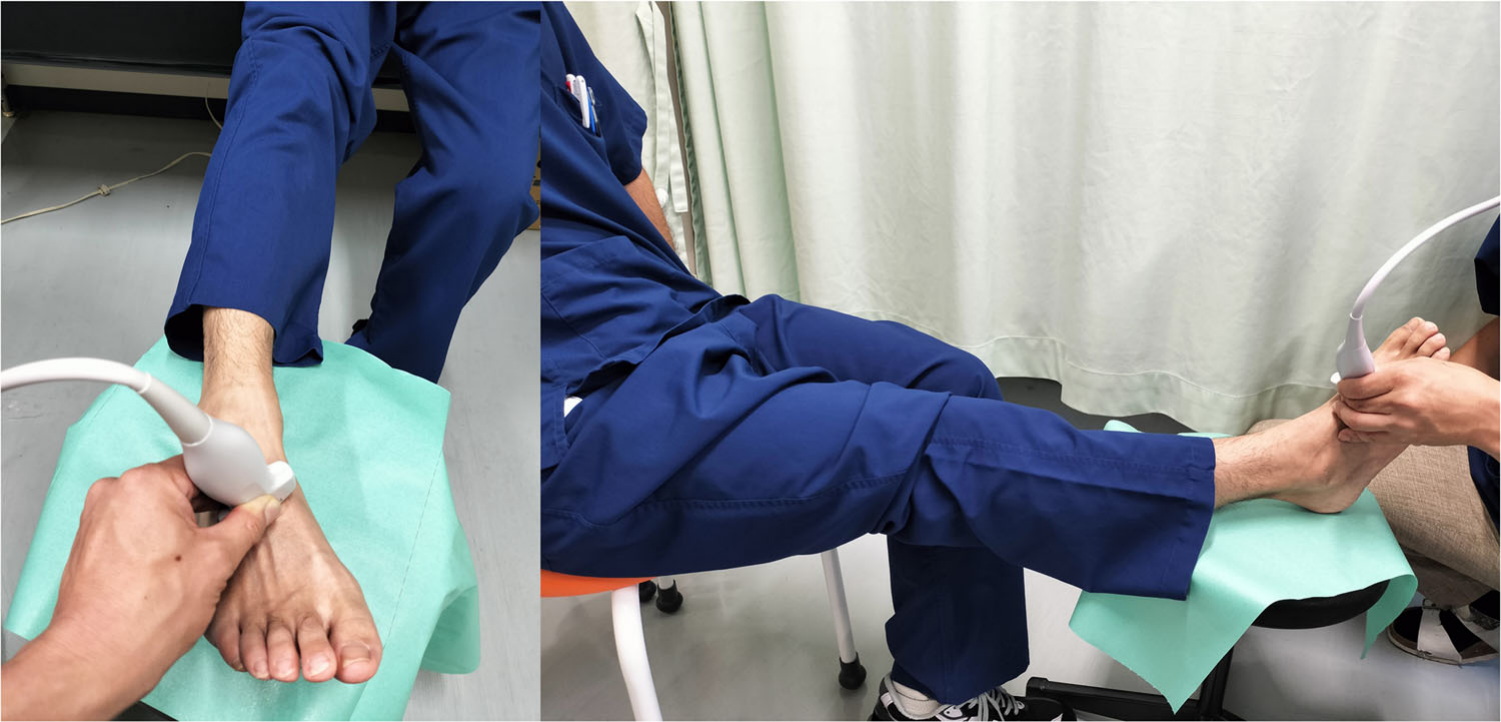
The ultrasound probe was placed on the dorsum of the C1 and M2 while the patients were in a sitting position, with hips flexed, knees extended, and ankles naturally plantar‐flexed.
